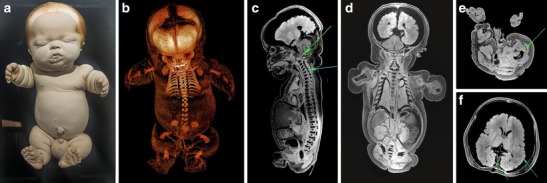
a Photograph of the full-term fetus of case 1. b Three-dimensional reconstructed skeleton based on the CT data. Although there was severely diminished bone calcification, CT images visualised extremely shortened ribs, short and small scapulae and iliac bones, and femoral and humeral bowing. c Sagittal T1-weighted MR image which showed a small foramen magnum with slight cranio-cervical caudal transition (*green arrow*), platyspondyly of the vertebra with short vertebral arches resulting in spinal canal stenosis (*turquoise arrow*). d Coronal T1-weighted MR images showed a severely hypoplastic thorax with presumably severe lung-hypoplasia. e Transverse T1-weighted MR image on the level of the femoral heads showed broad and irregular metaphyseal plates and extreme femoral bowing (*green arrow*) sometimes referred to as “telephone receiver” femora. f Transverse T1-weighted MR image of the brain which showed courser gyri of the temporal lobes (*turquoise arrow*) and excessive gyration of the occipital lobes (*green arrow*) and can be interpreted as polymicrogyria. Note the shrunken brain and small lungs which were both interpreted as normal post-mortem artefacts and probably strengthened by the formalin fixation. However, the presence of lung hypoplasia cannot be ruled out
